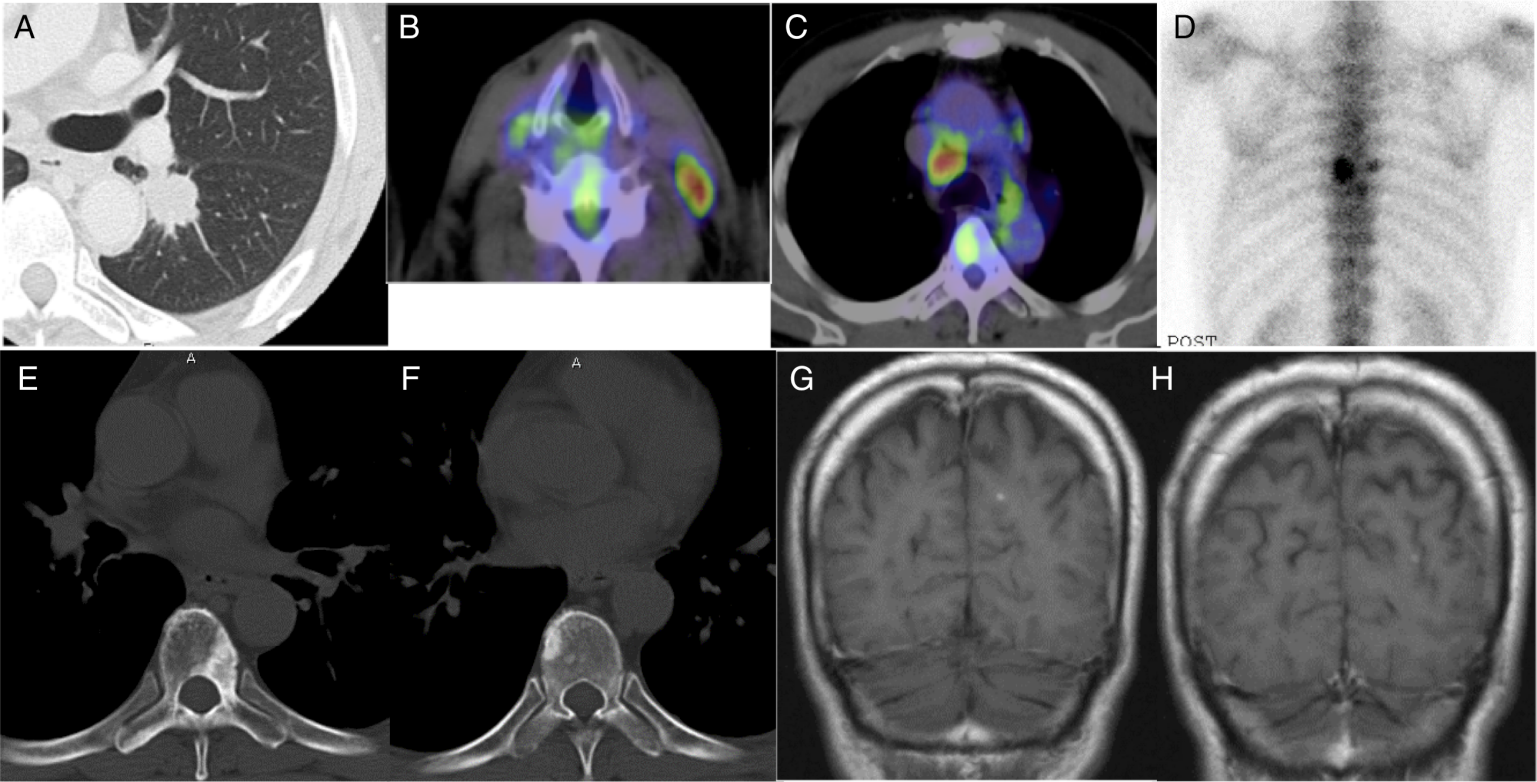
Images prior to commencing treatments: (A) Computed tomography (CT) demonstrated lung tumour of the left lower lobe. (B, C) positron emission tomography (PET)‐CT showed Fluorodeoxyglucose (FDG) uptake of left cervical and contralateral mediastinal lymph nodes. (D, E, F) Bone scintigraphy and CT demonstrated vertebral metastases. (G) Magnetic resonance imaging showed multiple small brain metastases.
